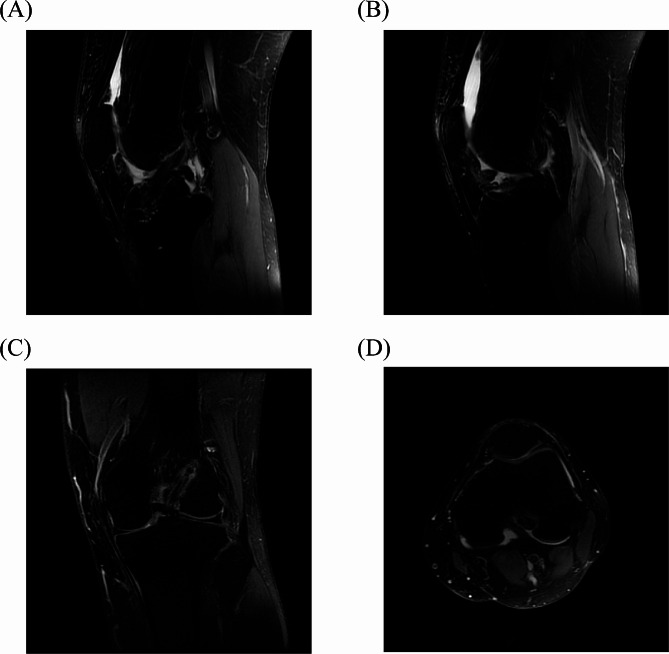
MRI images were reviewed 12 months after surgery. (A) Sagittal MRI showed good ACL continuity and good ligament tension after reconstruction; (B) Sagittal MRI showed the ligament at the femoral tunnel of ACL after reconstruction; (C) Coronal MRI showed the femoral intramuscular ligament of ACL after reconstruction; (D) MRI transverse section showed the ligament at the femoral tunnel of the ACL after reconstruction
